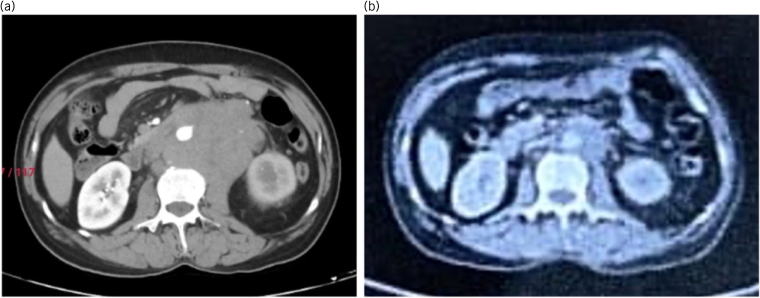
(a) Pre-chemotherapy contrast-enhanced computed tomography abdomen and pelvis scan showing a large retroperitoneal lymph nodal mass. (b) Post-chemotherapy positron emission tomography–computed tomography scan showing a significant reduction in the size of the retroperitoneal lymph nodal mass.
